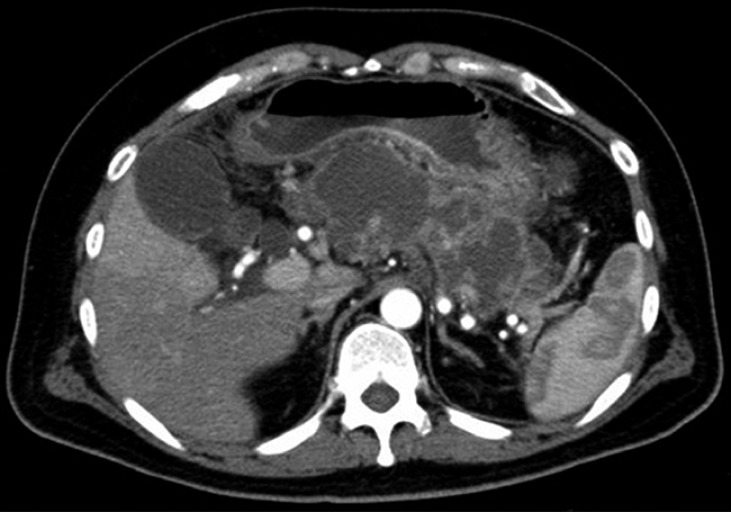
Computed tomography delineating a walled-off necrosis lesion. No abnormal vessels were observed along the possible puncture route of endoscopic ultrasound-guided puncture.
